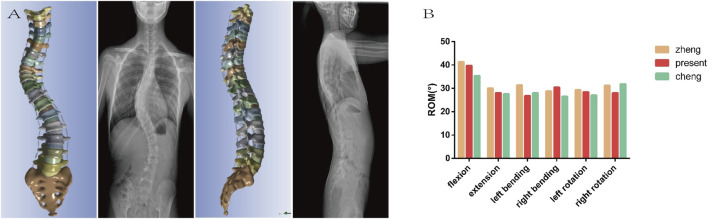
(A) The geometric validation indicates that the model is essentially consistent with the preoperative X-ray in both the coronal and sagittal planes. (B) The mechanical validation shows that the ROM of the model is basically between the values reported in the literature. Both of which concurrently confirms the effectiveness of the model.
